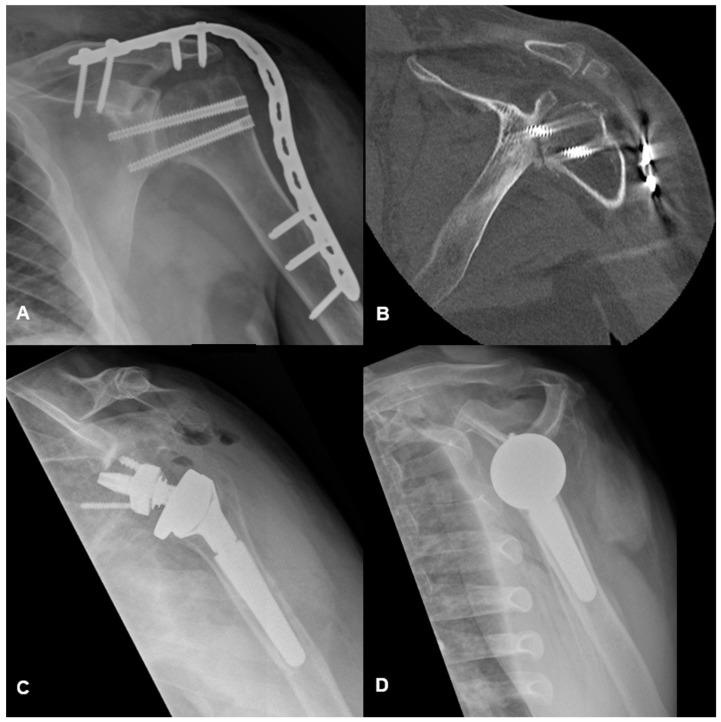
(A) shows an anteroposterior radiograph of the left shoulder of a patient with ongoing pain and mobility 4 months post-shoulder arthrodesis for osteoarthritis and recurrent seizures. (B) shows a post-operative axial computed tomography scan demonstrating lack of bridging callus formation. (C,D) are post-operative radiographs of the same patient undergoing reverse total shoulder arthroplasty after being seizure-free for 12 months.
